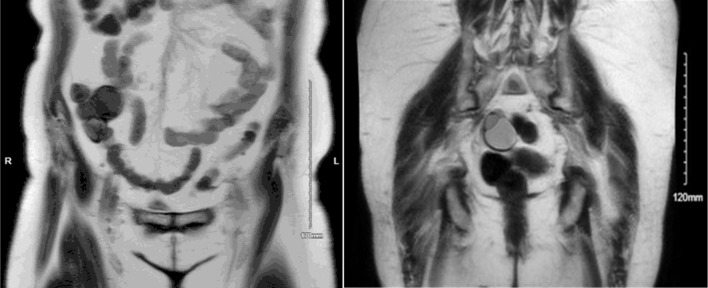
Coronal MRI images demonstrate an omental cystadenofibroma and an ovarian cystadenofibroma. The omental lesion appears as a well-circumscribed cystic mass within the anterior abdominal cavity, consistent with a benign omental implant, while the ovarian cystadenofibroma is located lower in the pelvis adjacent to the uterus, showing mixed cystic and solid components typical of epithelial origin. MRI: magnetic resonance imaging.
